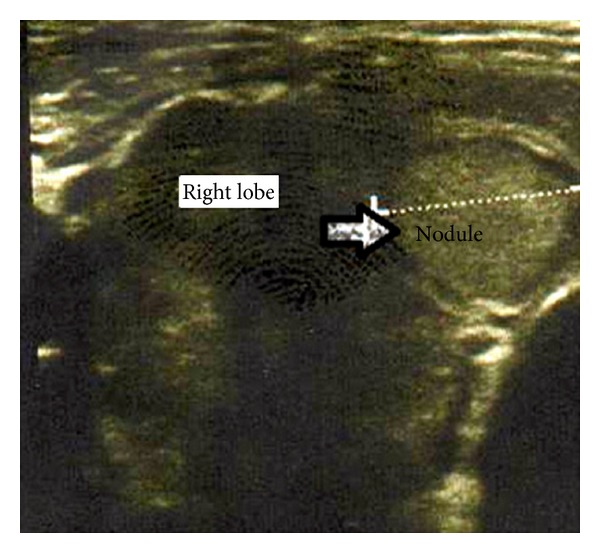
Ultrasonography of thyroid gland showing heterogeneous parenchyma and the solitary nodule in right lobe.
